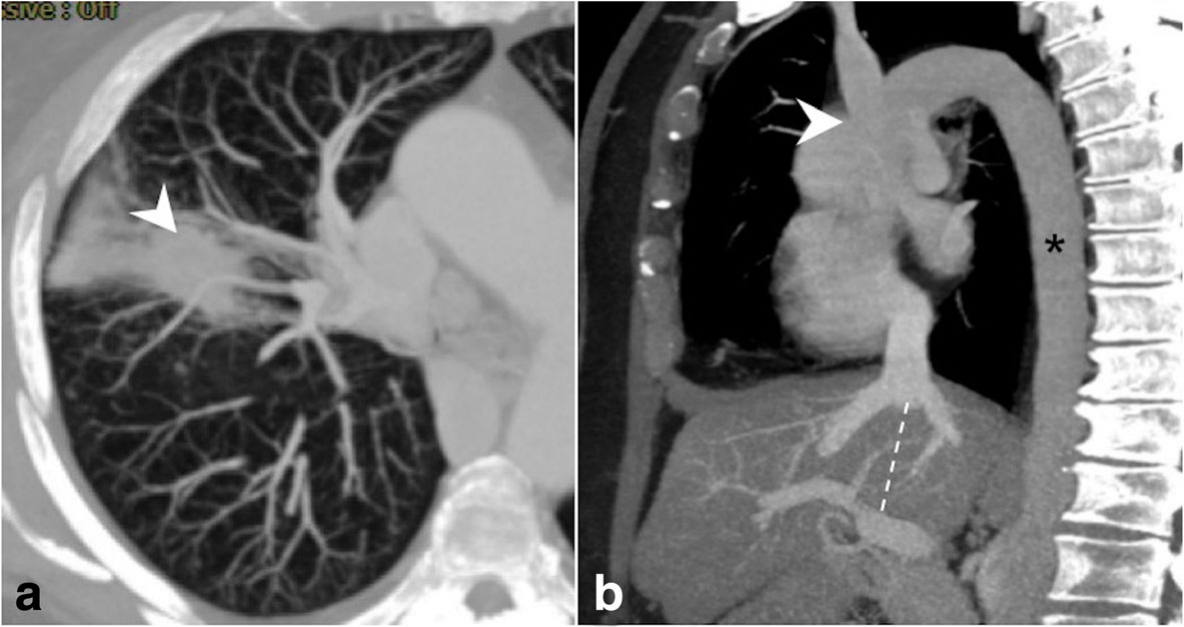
Preoperative CT scan showing right upper lobe squamous carcinoma (a, arrowhead). Multiplanar sagittal reconstruction shows enlarged azygos vein (b, asterisk) coursing through the diaphragm and draining into the superior vena cava (b, arrowhead); the hepatic inferior vena cava cannot be visualized along its normal course (b, dashed line). Findings are consistent with agenesis of the hepatic inferior vena cava and azygos continuation
